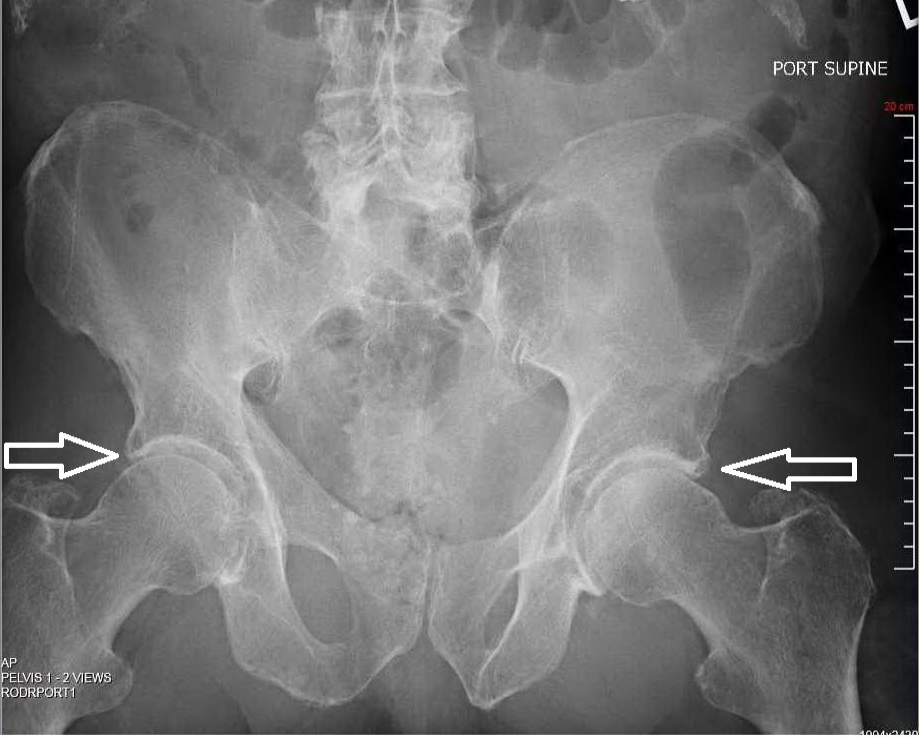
Anterior-posterior pelvic radiograph demonstrating the hips after reduction (arrows).
